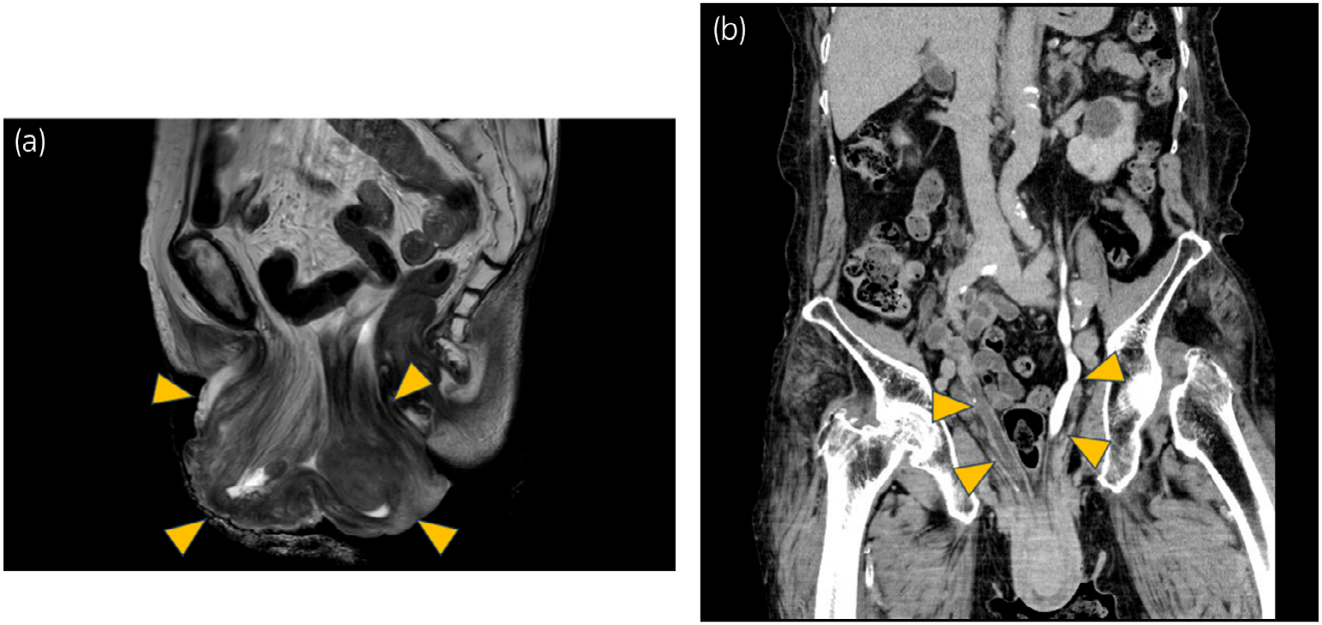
(a) MRI T2 sagittal view shows complete bladder and uterine prolapse. Arrows point to bladder and uterine prolapse. (b) The CT coronal section image shows the bilateral lower ureters were compressed at the pelvic floor and dilated proximally by the prolapsed bladder and uterus. The arrow points to the dilated ureter.
